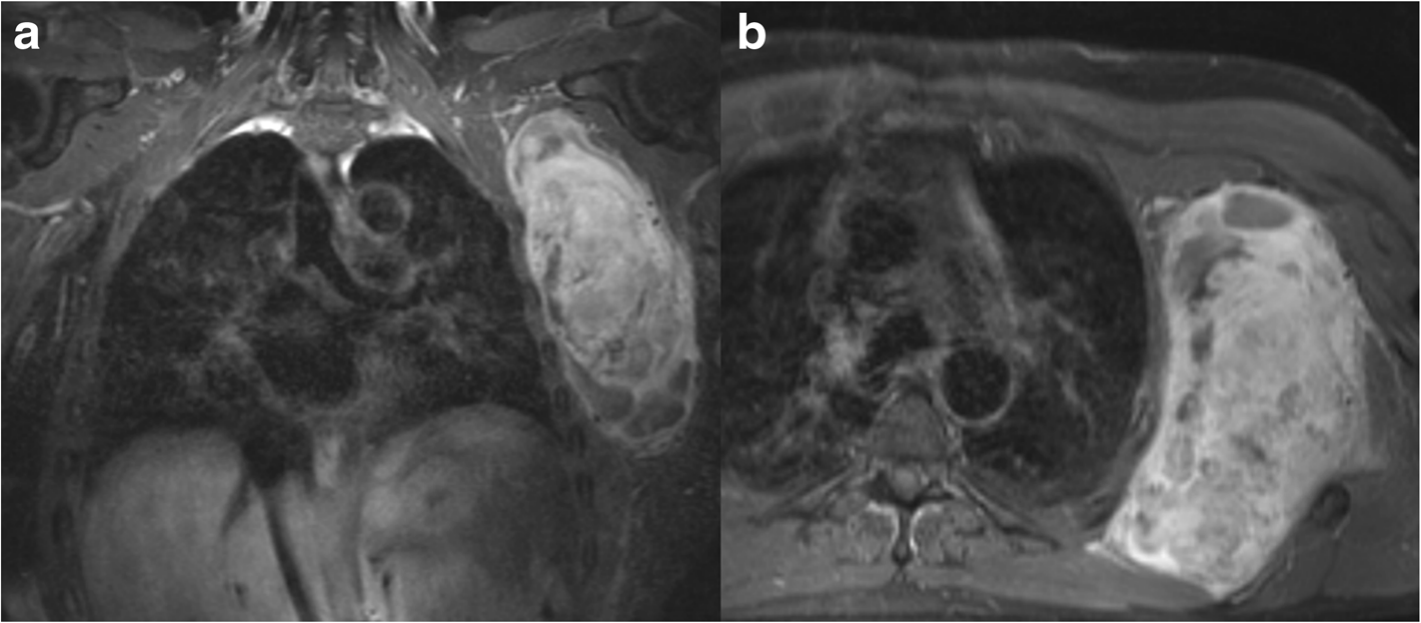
a, b MRI T1 with gadolinium enhancement (3a coronal plane/3b axial plane) imaging of a 77-year-old female with a radiotherapy-induced osteosarcoma of the glenoid (37 years after breast cancer treatment). The MRI shows the infiltration of the thoracic wall and a tumor encasement of the brachial vessel/nerve bundle
